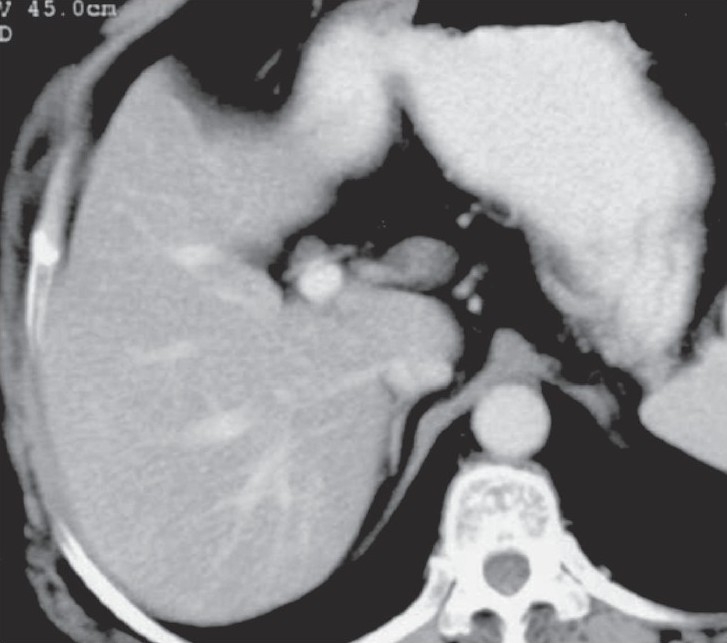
Axial contrast-enhanced CT scan of the liver done in May 2004 shows absence of focal hepatic lesions
